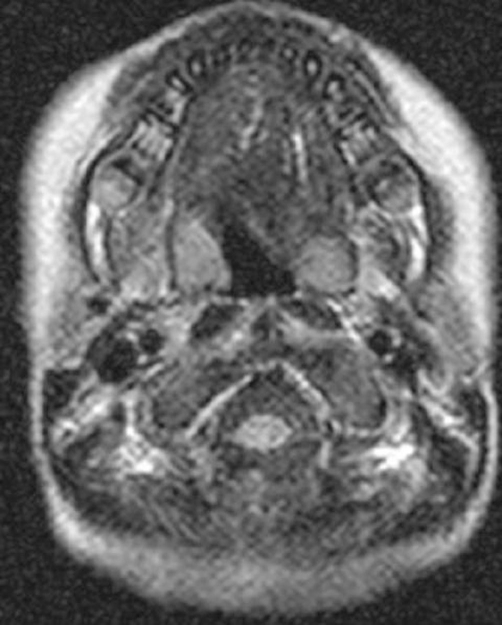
Axial MRI imaging of the craniocervical junction showed absence of the posterior arch of C1 (clefting).
